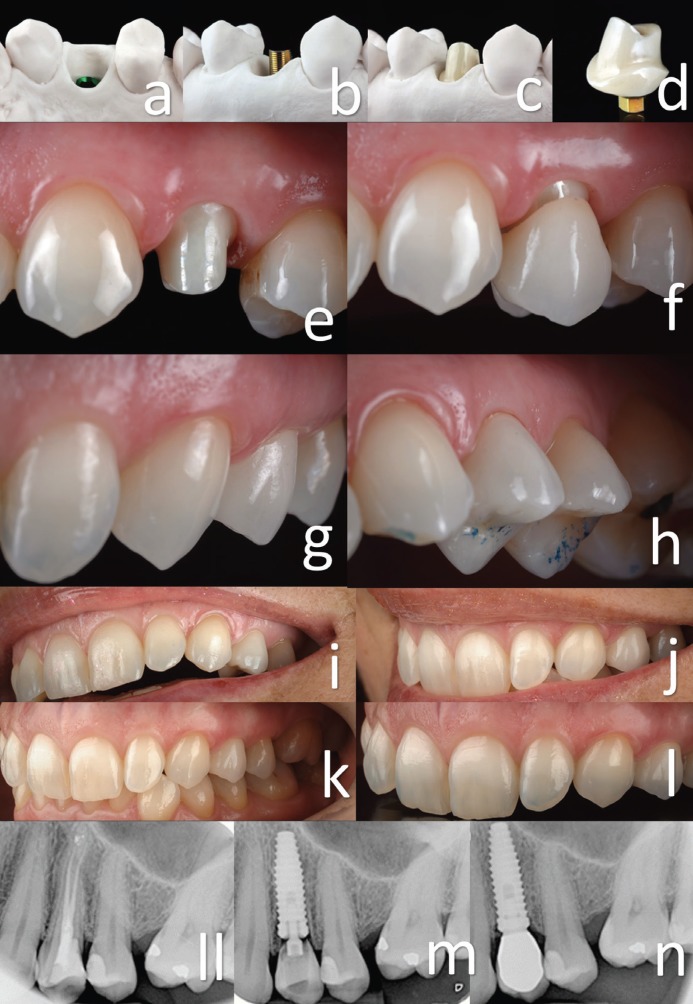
a) Cast with information transferred that faithfully reproduces the emergence profile. b) Testing the metallic interface on the implant analogue. c) Testing the zirconia abutment on the metallic interface. d) Zirconia abutment cemented on the metallic interface. e) Zirconia abutment test for the first time. f) Zirconia crown test for the first time. g) Harmonic results between the pink and white portions of the smile were achieved. h) Checking the occlusion. i, j, k, l) Final result achieved with only three appointments. ll, m, n)Radiographic sequence: initial situation, with the provisional, and with the final restoration.
